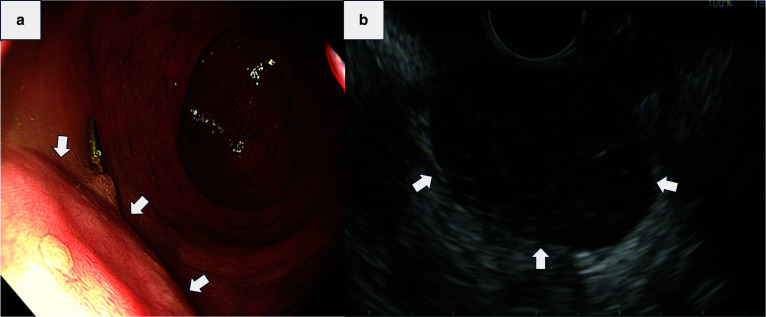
Colonoscopy and transrectal ultrasonography. a Colonoscopy showed a bulge that probably originated in the submucosal layer. b Transrectal ultrasonography showed a 28-mm submucosal tumor with clear borders and ring contouring
